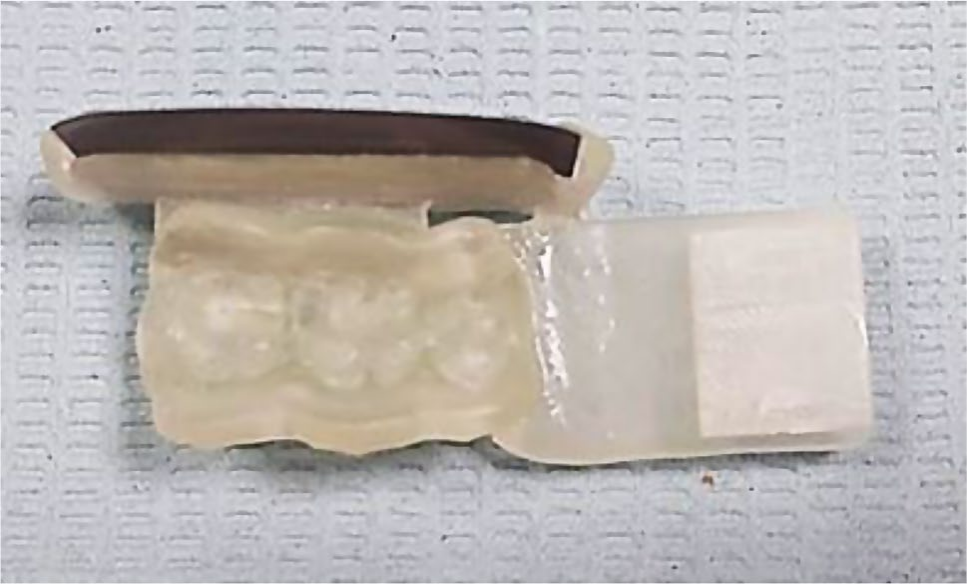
Customized radiographic template and film holder
